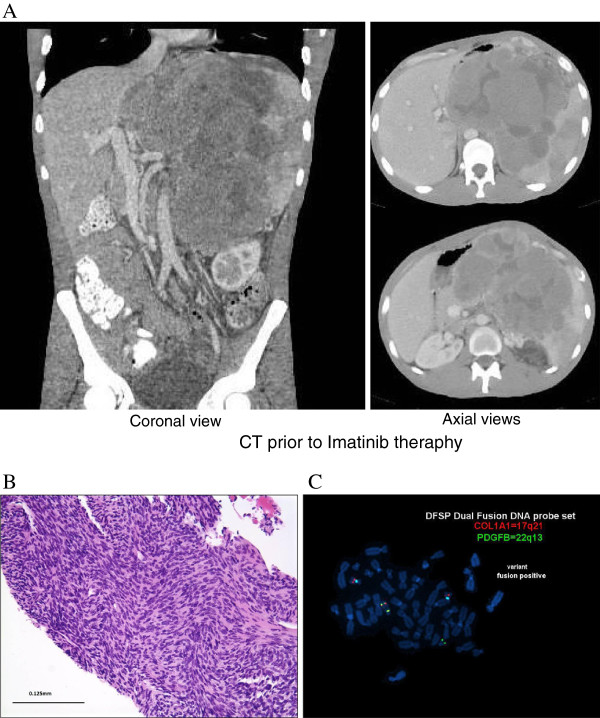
Radiographs of metastatic disease at diagnosis. A) CT scan demonstrating a large unresectable tumor involving all organs in the left upper abdomen. B) Biopsy of this mass showed malignant high grade spindled cells, C) a COL1A1-PDGFB translocation by dual fusion FISH probe set (yellow signals indicate the fusion signal), consistent with metastatic dermatofibrosarcoma protuberans with fibrosarcomatous transformation (DFSP-FS).
